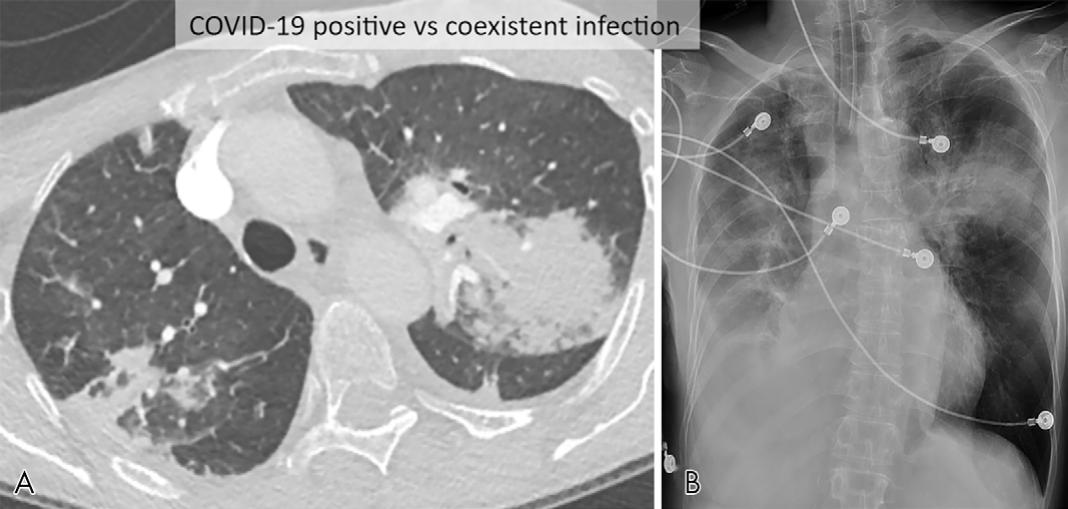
Atypical CT imaging features for COVID-19. Contrast-enhanced axial CT image (*A*) and frontal chest radiograph (*B*) showing segmental consolidation without significant GGO. Although this patient tested positive for COVID-19, the imaging features are not typical and could represent pneumonia related to COVID-19 or a secondary infectious process. Routine screening CT for diagnosis or exclusion of COVID-19 is currently not recommended by most professional organizations or the U.S. Centers for Disease Control and Prevention.
